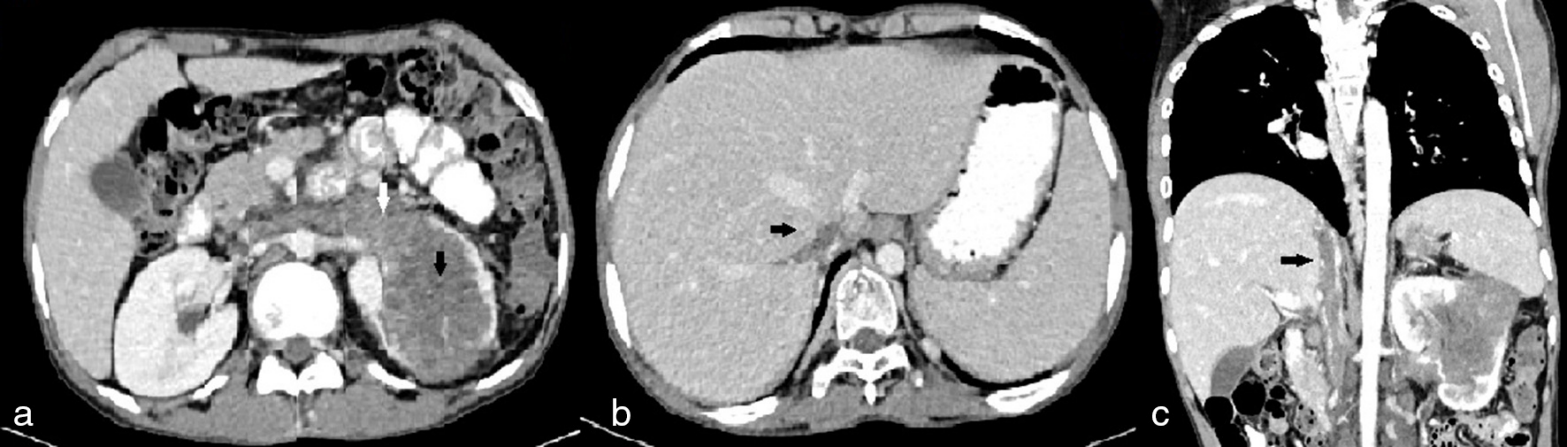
Contrast-enhanced CT scan of a 16-year-old male patient with renal Ewing’s sarcoma/primitive neuroectodermal tumour. (a) Axial section showing a large heterogeneous soft tissue mass (black arrow) arising from the left kidney having areas of necrosis within and extending into the renal vein (white arrow). (b) Axial section reveals the tumour thrombus extending into the inferior vena cava and right hepatic vein (black arrow). (c) Coronal reconstruction image demonstrating the left renal mass with tumour thrombus extending along the inferior vena cava (black arrow).
